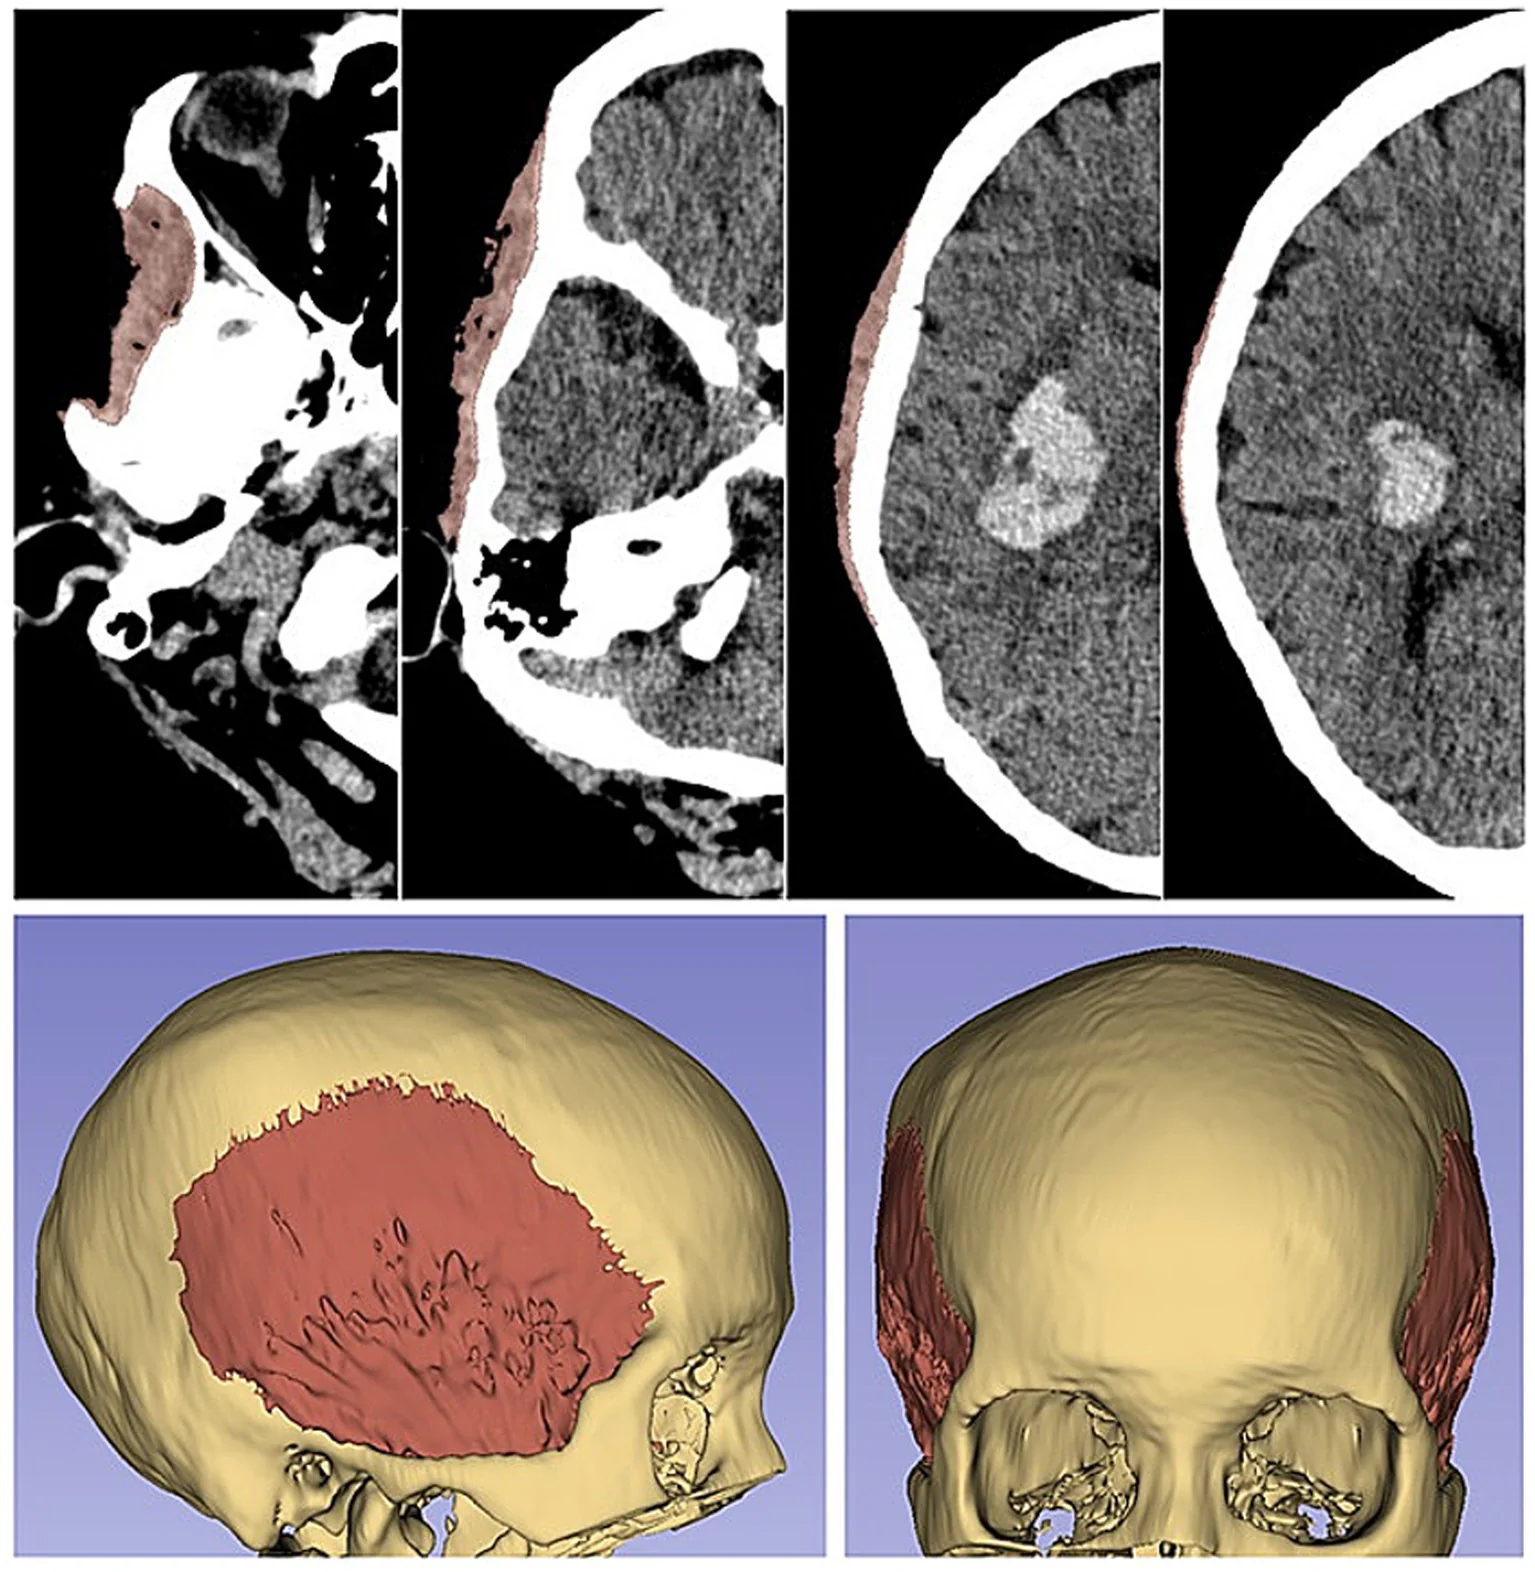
Representative CT images and three-dimensional reconstruction of the temporal muscles in a 62-year-old woman with hypertensive intracerebral hemorrhage. The hemorrhage was located in the right basal ganglia. The temporal muscles were manually segmented, as indicated by the red regions, and bilateral temporal muscle volumes were reconstructed and calculated using 3D Slicer software.
